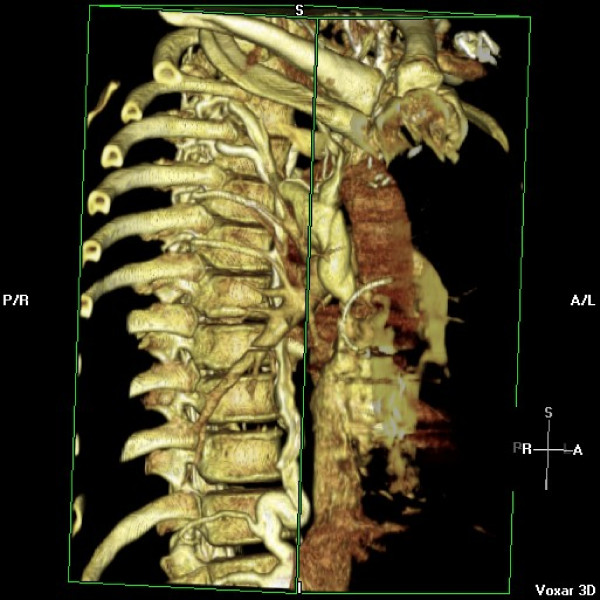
Contrast CT angiogram showing extensive backfilling of azygous system from superior vena cava.
